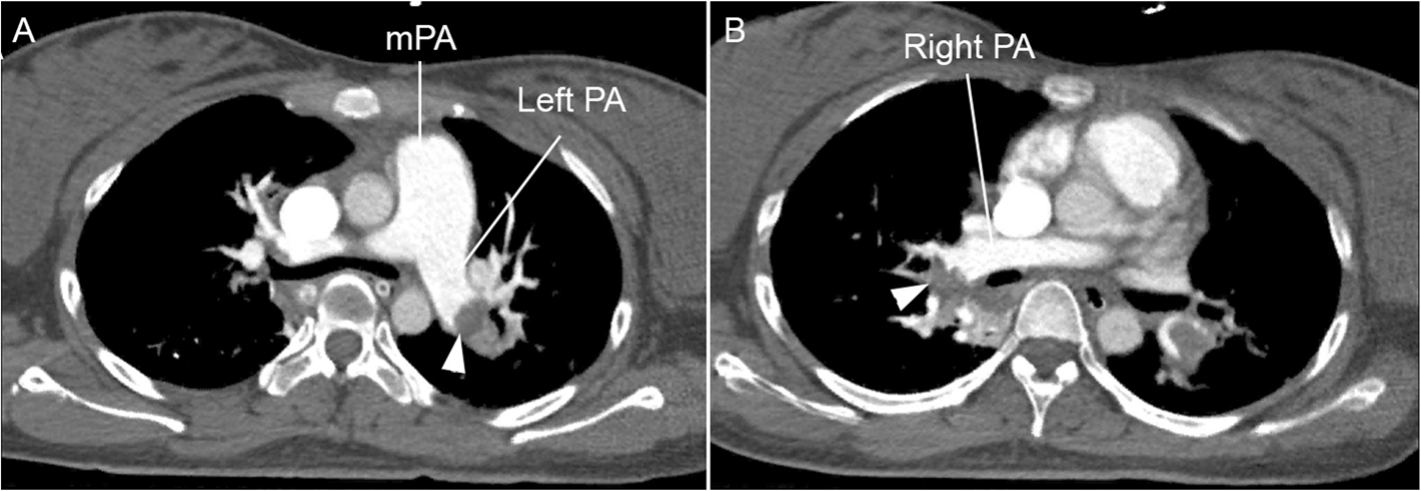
CTA of the chest (cross-section) shows large focal perfusion defects in the right a and right b pulmonary artery, representing bilateral pulmonary emboli
